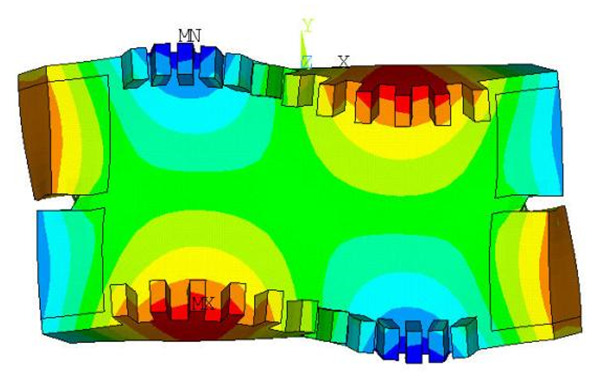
Working mode of the stator in the bidirectional self-propelled traveling wave linear ultrasonic motor.
